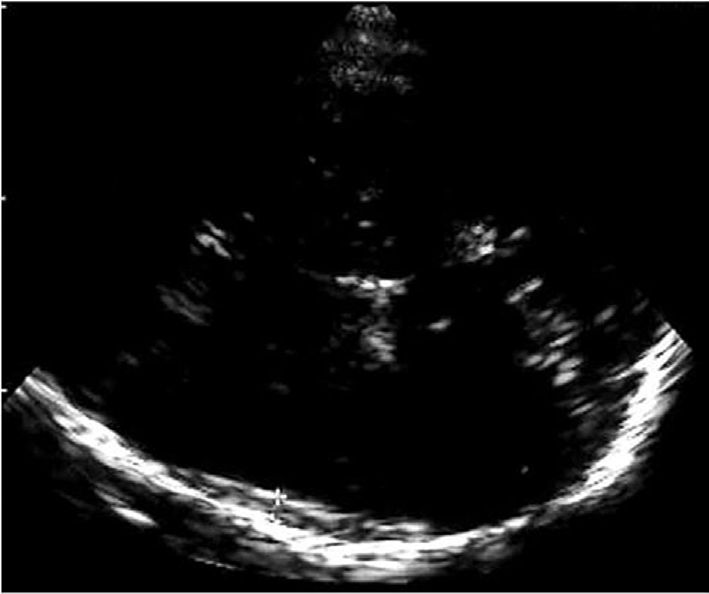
Figure shows the highly echogenic skull opposite to the probe and a highly echogenic membrane clearly distinctable from the skull as sonographic correlate of the dural border of the arachnoid and as a sign of subdural space enlargement.
